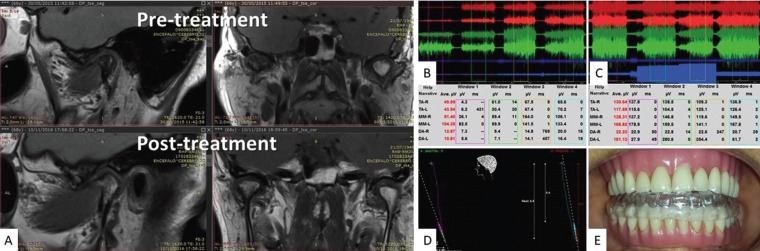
A) Upper images - Magnetic Nuclear Resonance (MRI) in PD (proton density) in slices (sagittal and coronal) in the pre-treatment, evidencing extensive bone marrow sclerosis in the right TMJ, facets, osteophyte and disc displacement with loss of the usual configuration. Lower images - MRI in PD (sagittal and coronal) in post-treatment control 18 months evidencing bone marrow area regeneration with hypersignal in the medullar of the right TMJ and increase of joint space by decompression. B) Joint decompression test before treatment (B) and post-treatment (C) with cotton rolls demonstrating maximum occlusion, right side roll occlusion, left side roll occlusion and bilaterally roll occlusion, In (B) with improvement muscle recruitment after increasing the vertical dimension. D) Take of the neurophysiological record from the mandibular three-dimensional / orthopedic positioning resulted from the neuromuscular deprogramming. E) view of occlusion with the use of the intraoral orthotic (IO).
